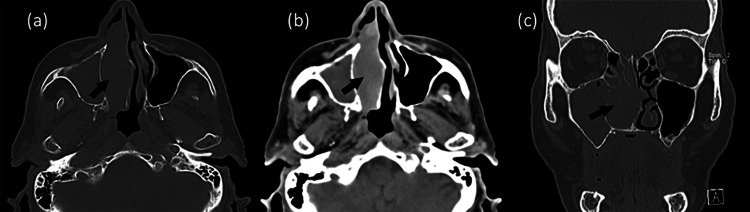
Computed Tomography (CT) of the nasal sinuses. Large mass deriving from the nasal septum, causing total obstruction of the right nasal cavity, chronic inflammation of the right maxillary sinus and right ethmoid cells and deviation of the nasal septum. Axial view: (a) and (b). Coronal view (c).
